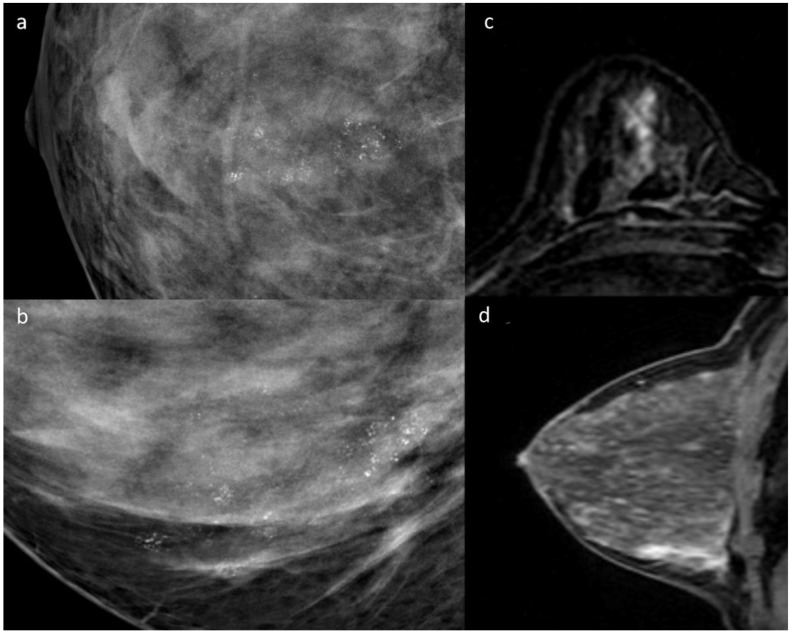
A 35-year-old female with biopsy result B3—ADH. Mammography shows the presence of amorphous microcalcifications with regional distributions at the union of the lower quadrants (a,b). DCE-MRI shows linear heterogeneous non-mass enhancement (c,d).
